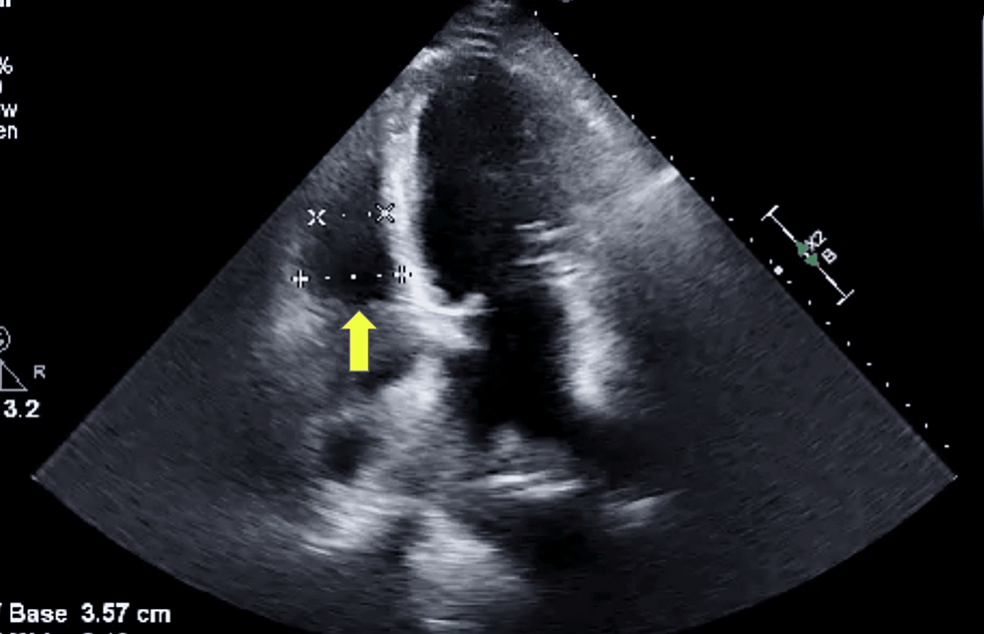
Echocardiography showing moderately dilated right ventricle (yellow arrow)
